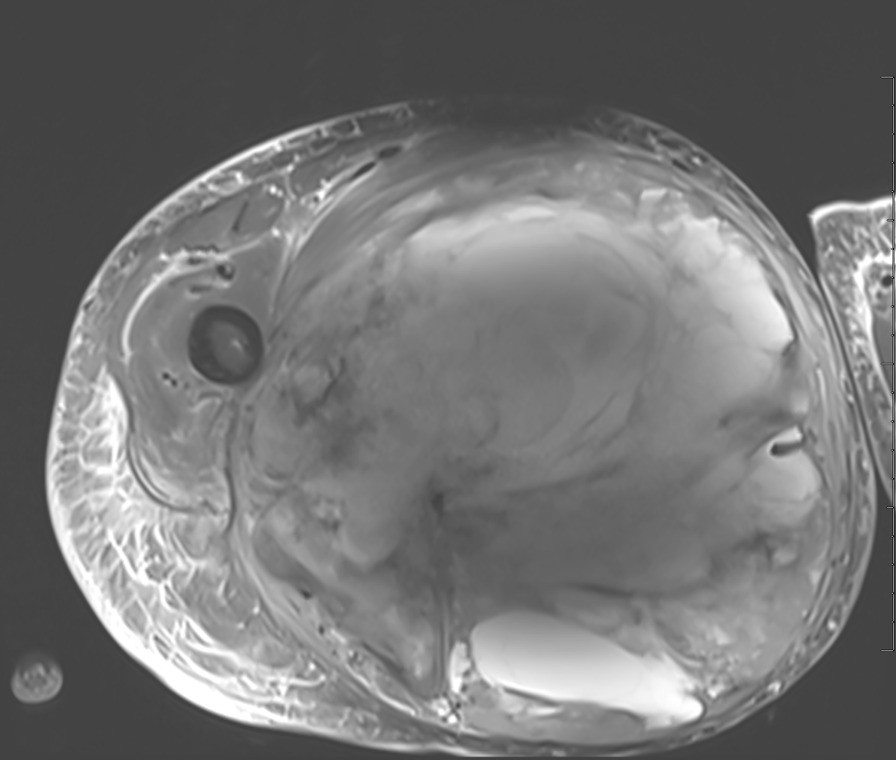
Post-treatment MRI images. Axial PD fat suppressed MR image of the right thigh demonstrates interval increase in the size of the soft tissue mass, measuring 21.6 × 20.9 cm in transverse and AP dimension
